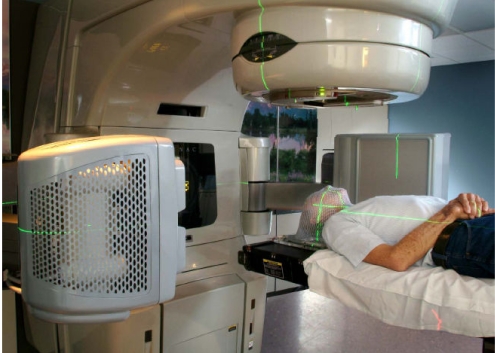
A linear accelerator with built-in kV cone beam CT system for IGRT treatment delivery (courtesy of Varian Medical Systems)
